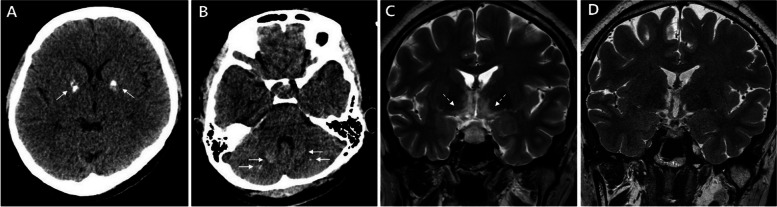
A, B Non-contrast enhanced CT showing calcifications in the bilateral basal ganglia and cerebellum (arrows). C T2-weighted image showing a pituitary mass with infiltrative T2 hyperintense lesions in the hypothalamus, optic chiasm and tracts, and thalamus (dashed arrows). D Follow-up MRI performed 8 weeks later showing a marked improvement
